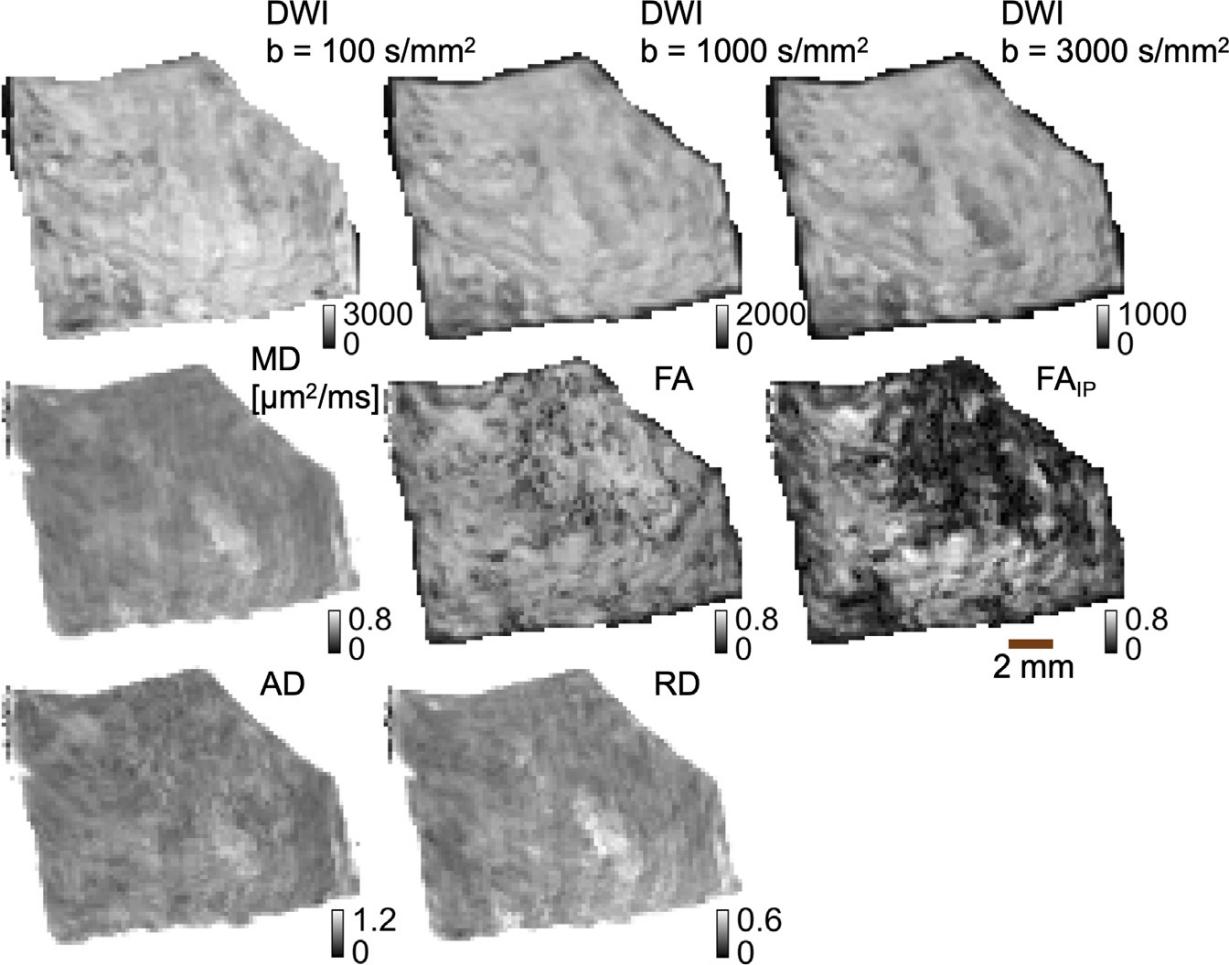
Overview of the DTI modalities in sample 5. In the upper row, the DWI signal averaged across all directions for the given b-value is shown. In the middle, row mean diffusivity (MD), fractional anisotropy (FA), and in-plane fractional anisotropy (FA_IP_) are visualized. In the bottom row, axial diffusivity (AD) and radial diffusivity (RD) are shown. Not shown are the eigenvalues of the diffusion tensor (J_11_, J_12_, J_22_).
